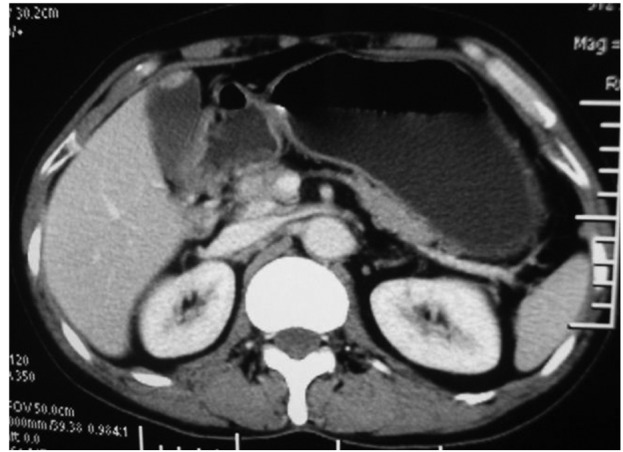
Abdominal computed tomography (CT) showing a regular intraluminal polypoid mass in the gallbladder.
